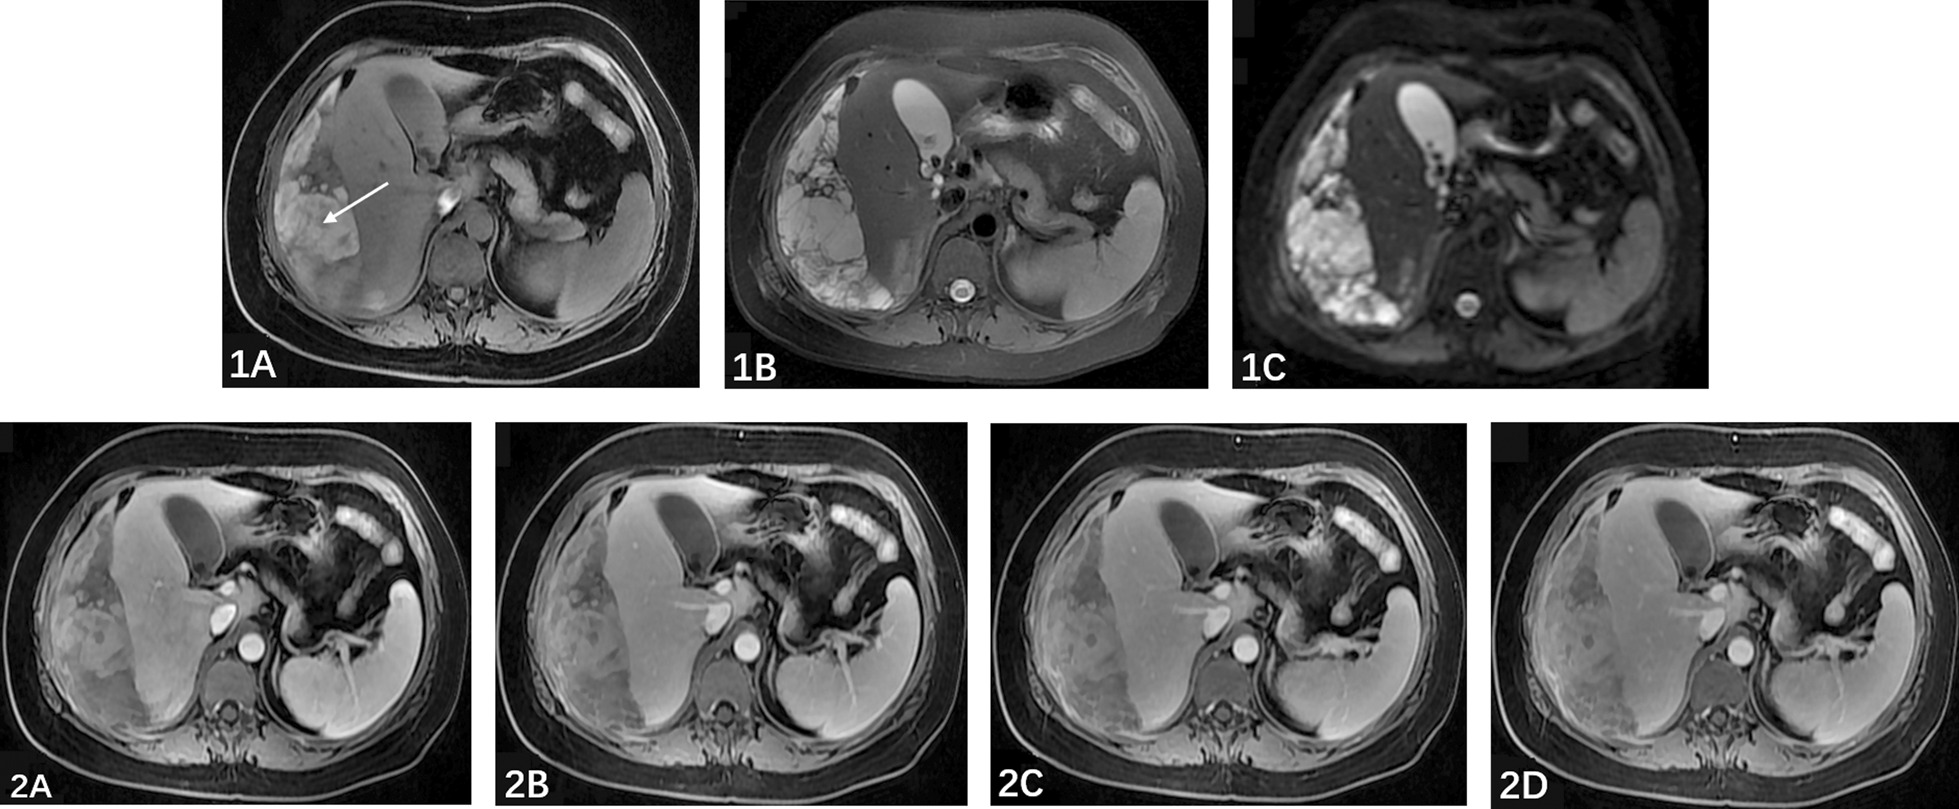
(2019-02-15) Abdominal MRI: an irregular mass with mixed short T1-weighted imaging (T1WI), mixed long T2-weighted imaging (T2WI) and high diffusion-weighted imaging (DWI) under the capsule of the right lobe of the liver. After enhancement, the mass showed uneven enhancement. (1A: T1WI sequence; 1B: T2WI sequence; 1C: DWI sequence; 2A: Arterial phase; 2B: Portal phase; 2C: venous phase; 2D: delayed phase.)
